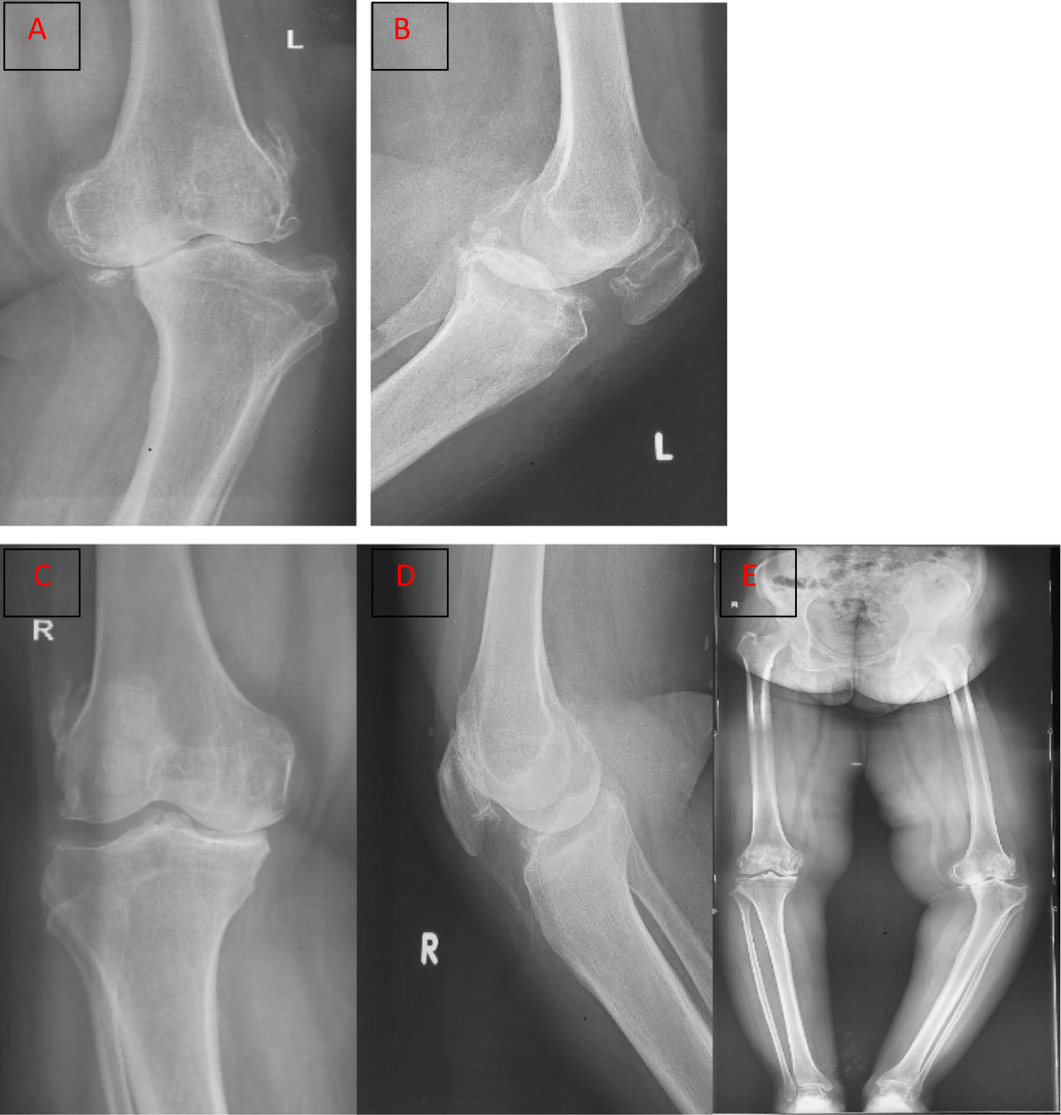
a. Anteroposterior (AP) X-ray of left knee showing advanced degenerative changes and severe varus deformity. b Advanced degenerative changes on lateral view. c Degenerative changes on AP view of right knee. d Lateral view of right knee. e Full-length standing scanogram showing bilateral varus deformities, which were more severe on the left side
